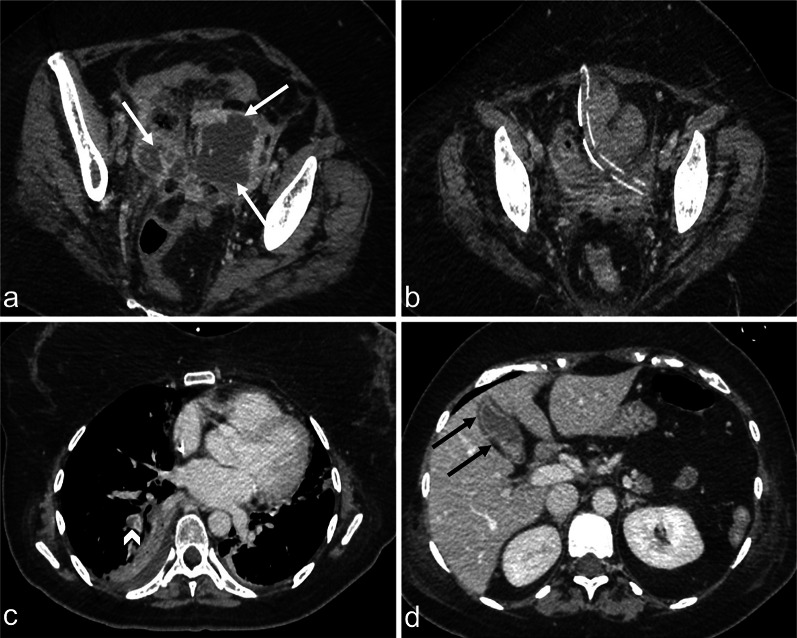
Contrast-enhanced CT image in the portal venous phase showing a 44-year-old female with abscessed uterine neoplasm (a, arrows) and post-operative control with completely drained collection (b). Pulmonary thromboembolism (c, arrowhead) with development of a clinical septic state (qSOFA 2). Note the dense, gallbladder mural enhancement without thickened walls (d, black arrows)
